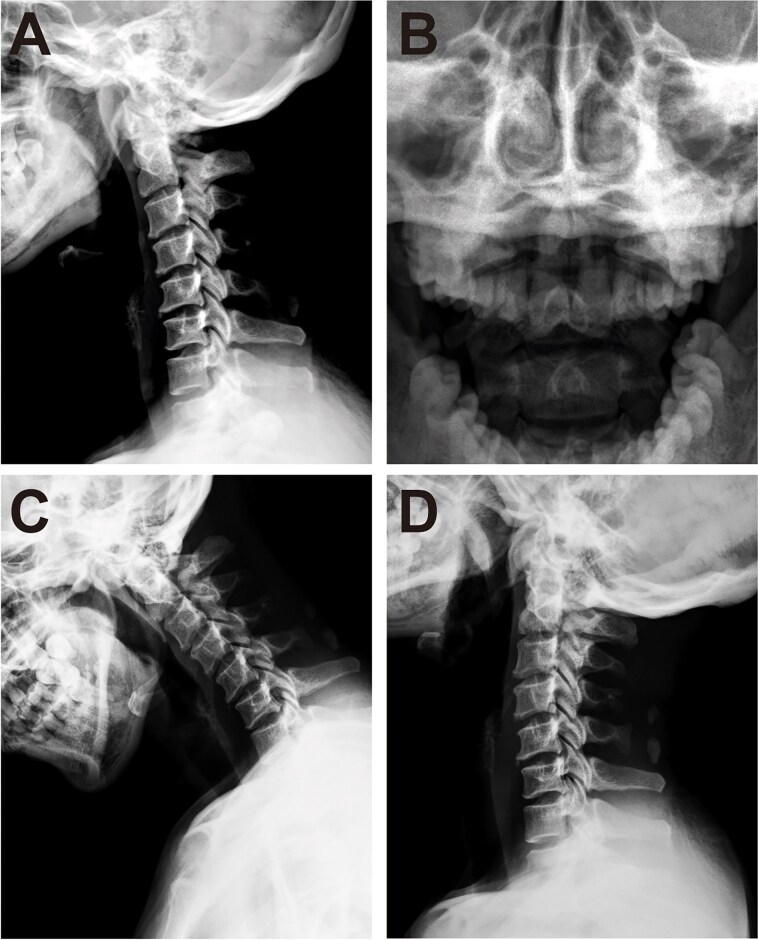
Cervical spine radiograph. (A) Lateral radiograph demonstrating partial aplasia of the posterior arch of the atlas. (B) Open-mouth radiograph showing no atlantoaxial dislocation. (C, D) Cervical spine dynamic X-ray.
